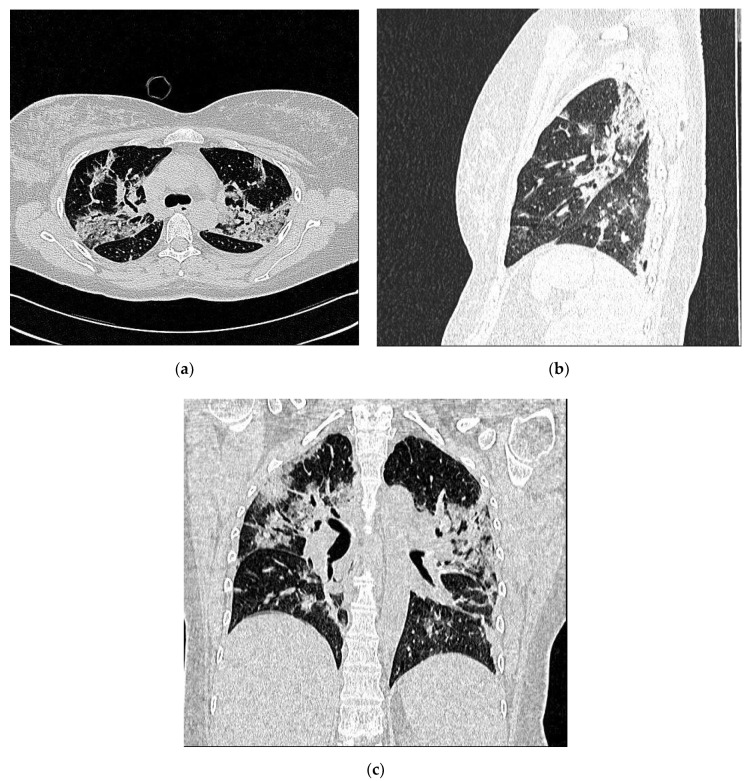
CHEST CT shows extensive interstitial pneumonia on the axial plane (a) with crazy paving pattern and consolidation area. Multiplanar reconstruction (MPR) on coronal and sagittal planes (b,c) demonstrate scissure thickening and fibrosis.
